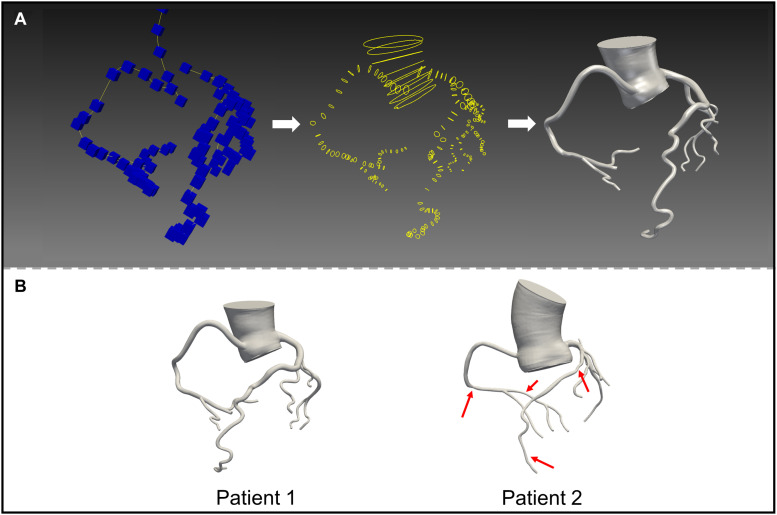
3D reconstructed mode ls based on computed tomography images. (A) Construct the centerlines of the vessels (left); segment the vessel perpendicular to the centerline (middle); loft the segmentations (right). (B) The models of patient 1 and 2.
